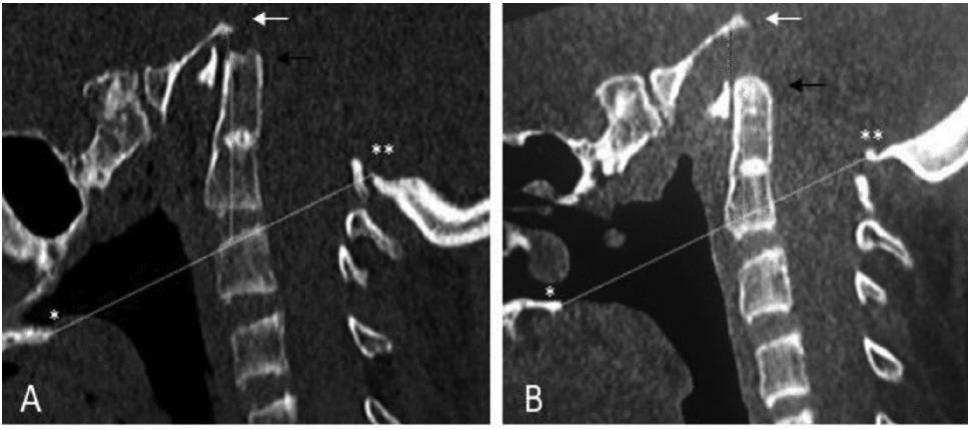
Pre-and postoperative BI measures on sagittal CT scan: the Chamberlain’s line (yellow line) is drawn from the posterior surface of the hard palate (*) to the tip of the Opisthion (). The basilar-dens interval (red line), measured from the Basion (white arrow) to the tip of the dens (black arrow). A CT scan of CVJ before Halo-traction: the tip of the dens (black arrow) is placed 30 mm above this line while the basilar-dens interval was 3 mm. B CT scan of CVJ after 6 weeks of treatment by Halo-traction: the tip of the dens was placed 20 mm above the Chamberlain line, with a basilar-dens interval passing from 3 to 9 mm
